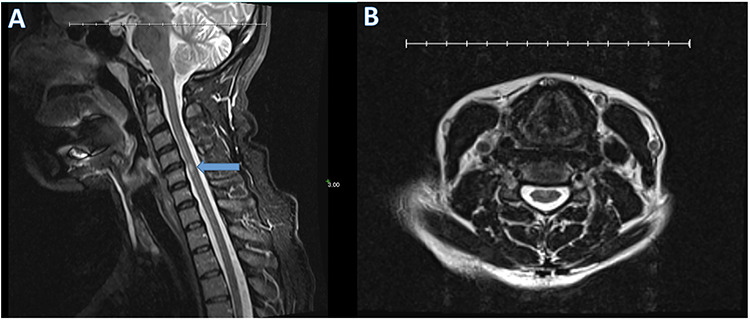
T2-weighted sagittal (A) and axial (B) cervical spine MRI reveals a 4 mm hyperintense area within the spinal cord at the C4–C5 level (arrow) and a disc bulge without spinal cord deformity.
